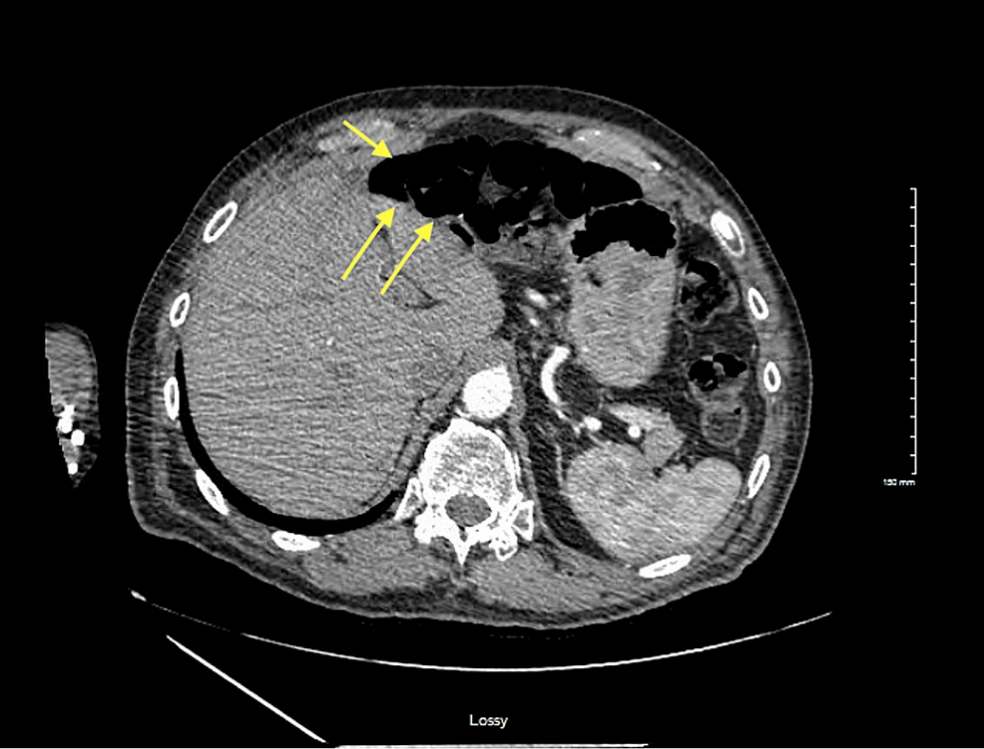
CT angiogram of the abdomen/pelvis Abdominal CT angiogram reveals the bowel loops receding and not interposed between the liver and abdominal wall CT: computed tomography
